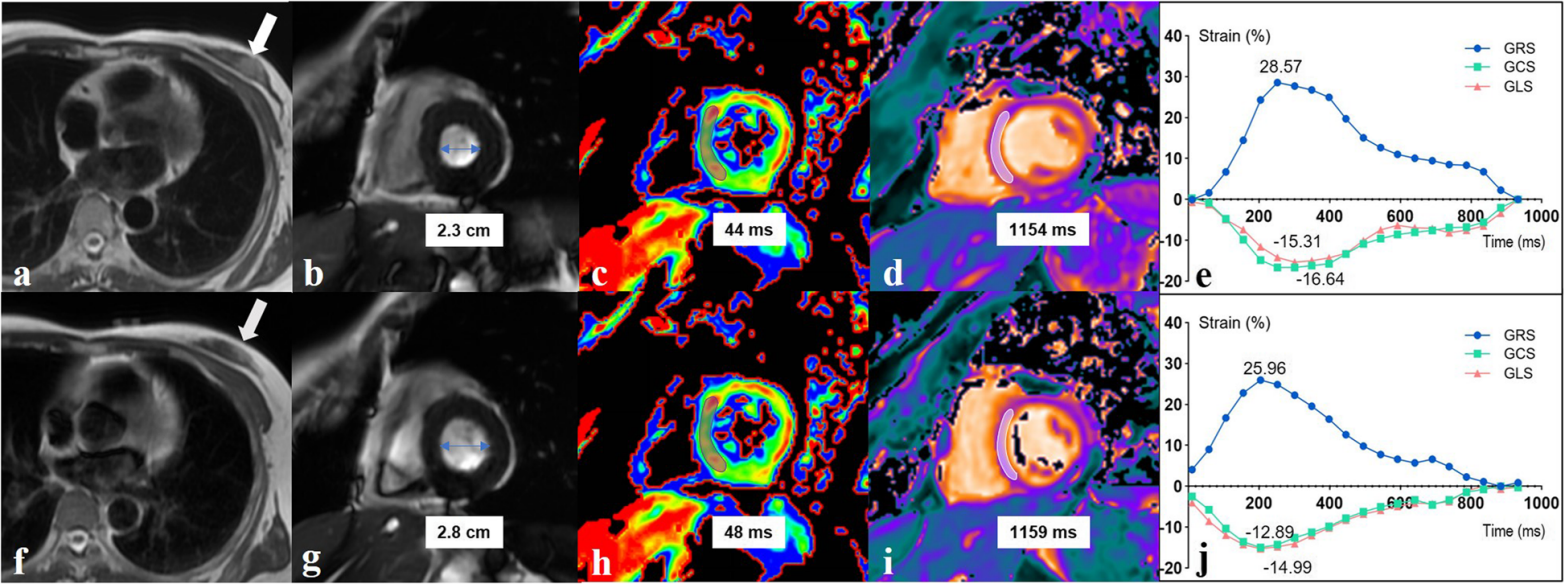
Cardiac magnetic resonance imaging in a 59-year-old woman with human epidermal growth factor receptor 2 positive left-side breast cancer. Left ventricular ejection fraction was 66% at baseline and 56% at follow-up. Top images (a–e) are at baseline. Bottom images (f–j) are after four cycles of the taxanes plus carboplatin plus trastuzumab plus pertuzumab regimen (95 days after the baseline scan). Axial T2-weighted images (a, f) show a decrease in the tumour size of the left breast (arrows). Short-axis cine images (b, g) demonstrate an increase in left ventricular end-systolic internal diameter (2.3 cm at baseline, 48 cm at follow-up). T2 mapping images (c, h) show a slight increase in T2 relaxation time in the septum of the mid short-axis slice (44 ms at baseline, 48 ms at follow-up). T1 mapping images (d, i) show a slight increase in T1 relaxation time in the septum of the mid short-axis slice (1,154 ms at baseline, 1,195 ms at follow-up). Global radial, circumferential and longitudinal strains before and after four cycles of therapy (e, j). *GCS* Global circumferential strain, *GLS* Global longitudinal strain, *GRS* Global radial strain
